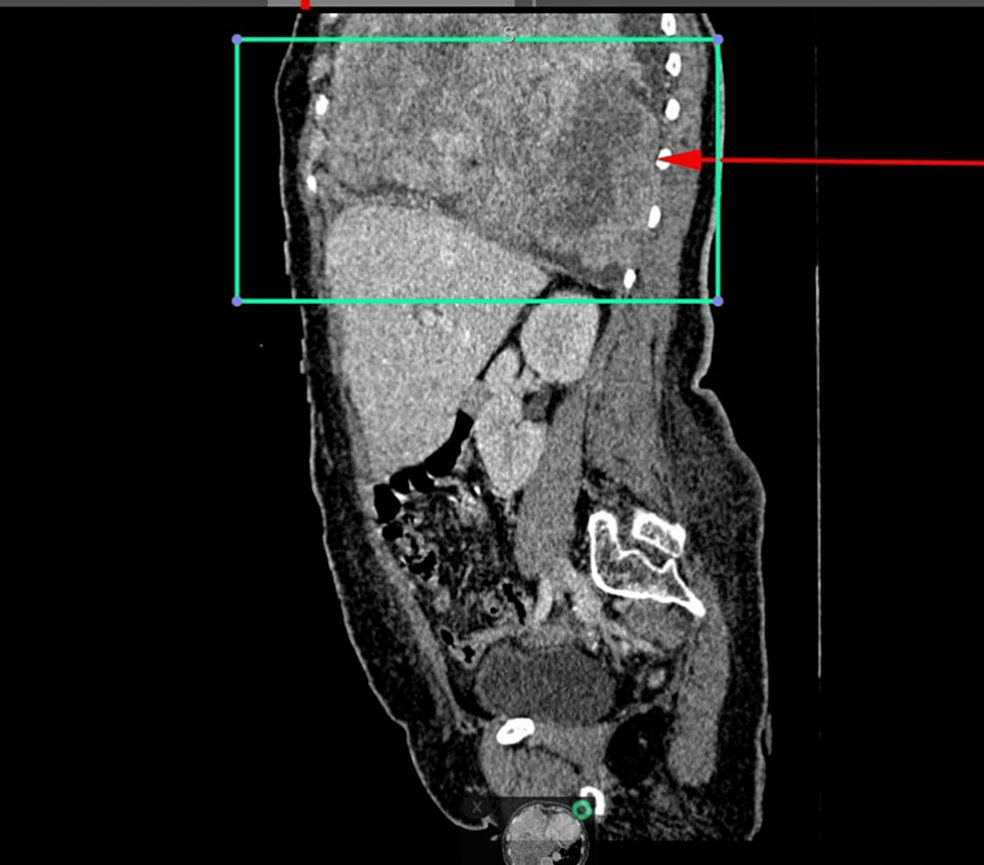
CT sagittal IV contrast demonstrates the large lesion limited by the diaphragm with no evidence of intraabdominal extension. CT: computed tomography; IV: intravenous
